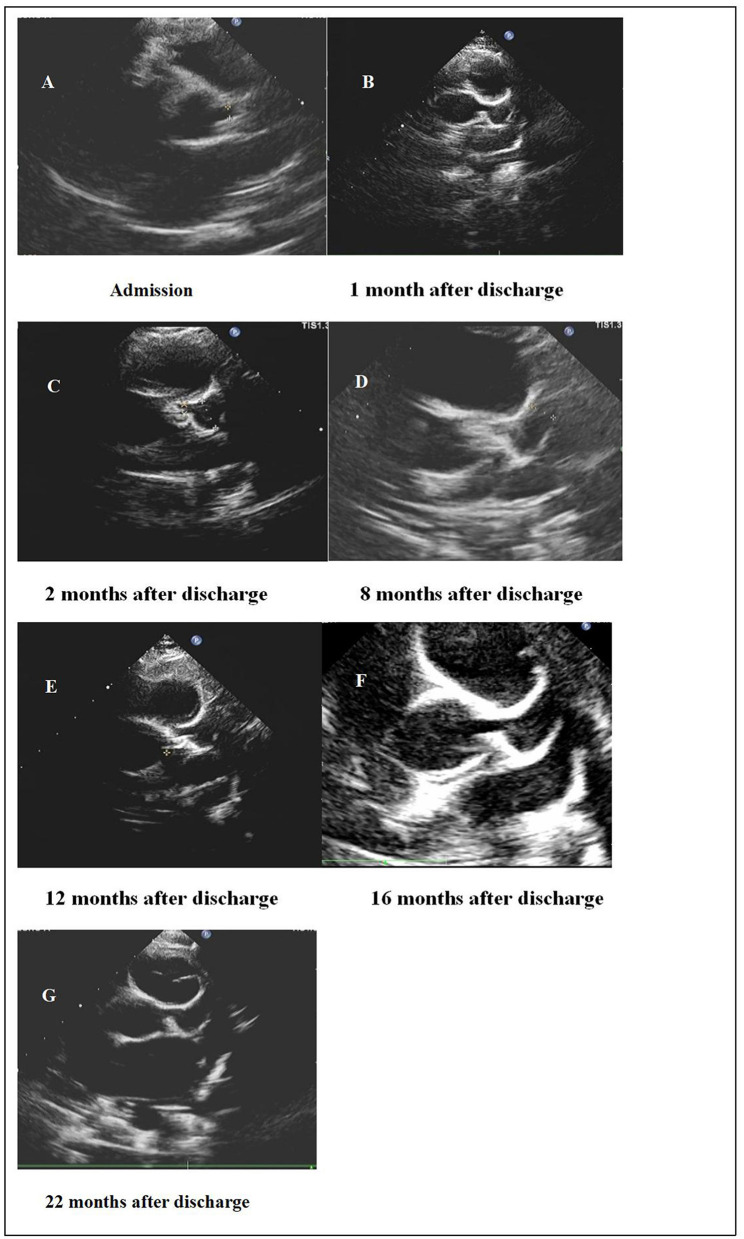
Dilation of the left coronary artery showed by cardiac color Doppler ultrasound.
